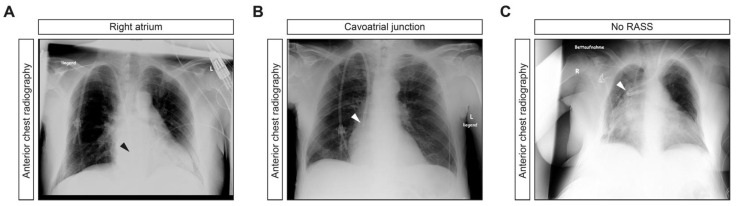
Portable anterior-posterior chest radiography. (A,B) Representative radiographic images of proper HDC placement, with tip position in the right atrium or cavoatrial junction (arrowheads). (C) Radiographic image of improper HDC placement, with kinking at the subclavian-caval junction, due to central vein stenosis (arrowhead). Abbreviations: HDC, hemodialysis catheter; RASS, rapid atrial swirl sign.
